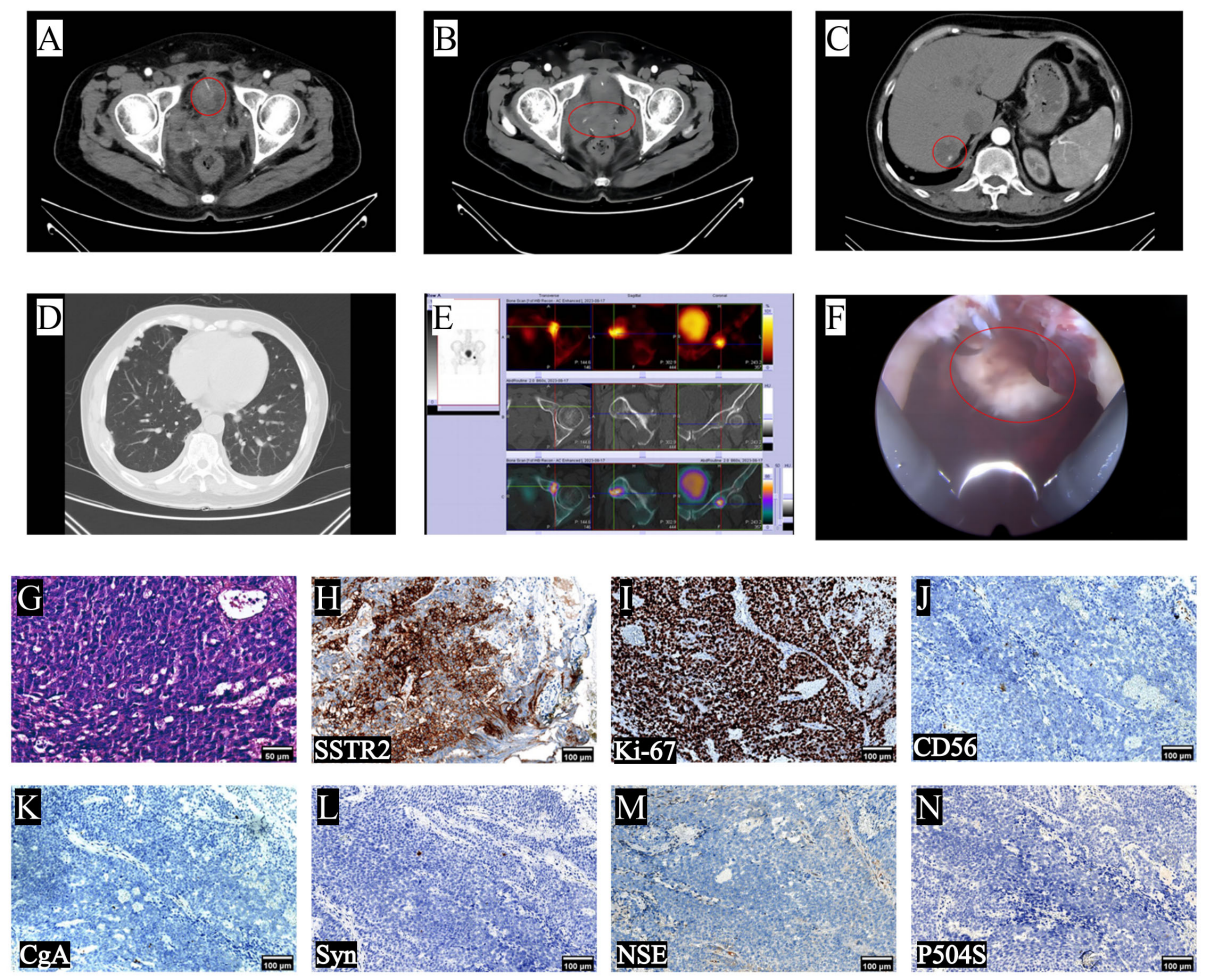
Imaging changes after patient progression, intraoperative conditions and postoperative findings. (A, B) CTU scans revealed the recurrence of bladder and pelvic tumors; (C) upper abdomen CT indicated hepatic metastasis; (D) chest CT showed multiple metastases; (E) WBBS indicated bone metastases; (F) intraoperative cystoscopy reveals tumor at 12 o’clock in the bladder; (G) typical LCNEPC presentation, no adenocarcinoma component, IHC was positive for (H) SSTR2 and (I) Ki-67 (90%), and negative for (J) CD56, (K) CgA, (L) Syn, (M) NSE and (N) P504S (10×).
